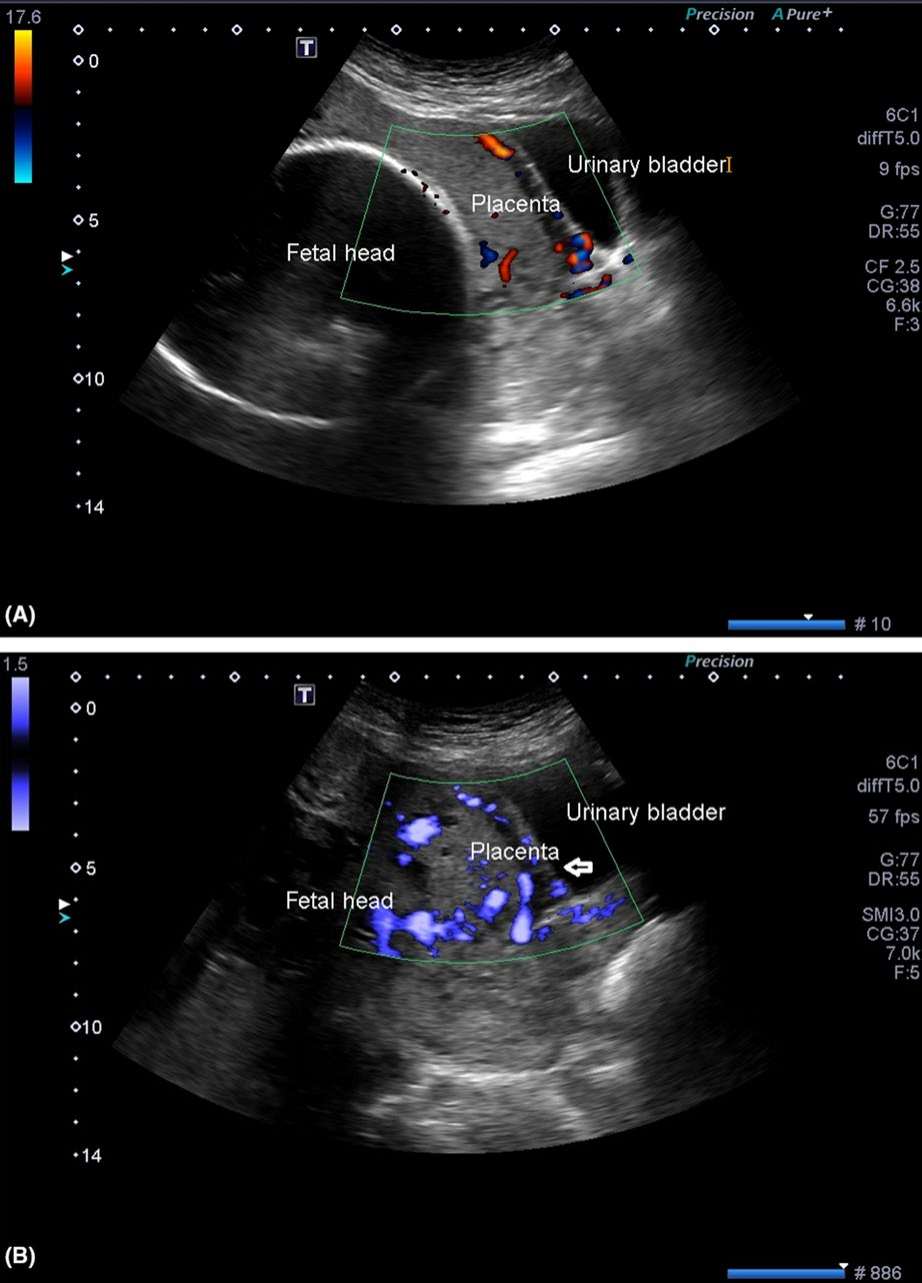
Sagittal transvaginal ultrasound image shows (A) Thinning of the myometrium in the lower uterine segment with few prominent vessels along the scar line. (B) The placenta is seen extending to the serosal surface of the bladder
